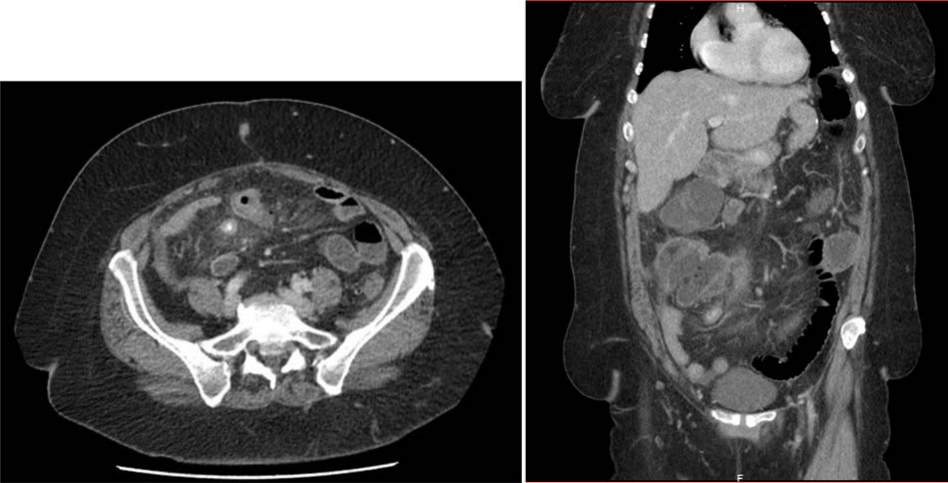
Emergency CT: acute appendicitis with: intra-appendicular liquid, microstercoliths and peri-appendicular inflammation.
